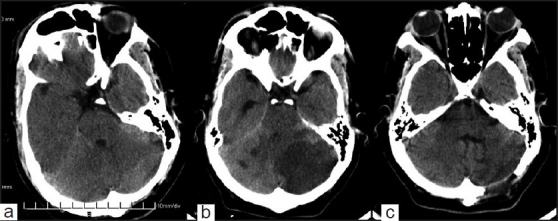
(a) CT scan showing right cerebellar infarction, (b) follow-up CT scan showing infarction, and (c) postoperative scan showing the opened up ventricle
